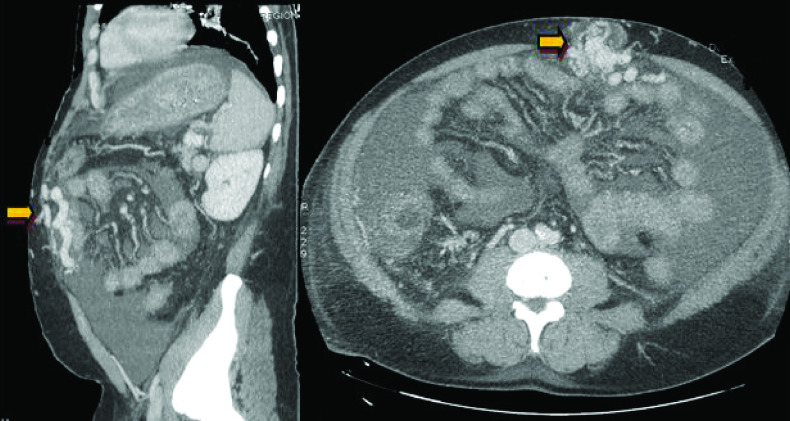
Computed tomography images showing a parietal varicose veins secondary to the permeabilization of the umbilical vein (yellow arrow).
